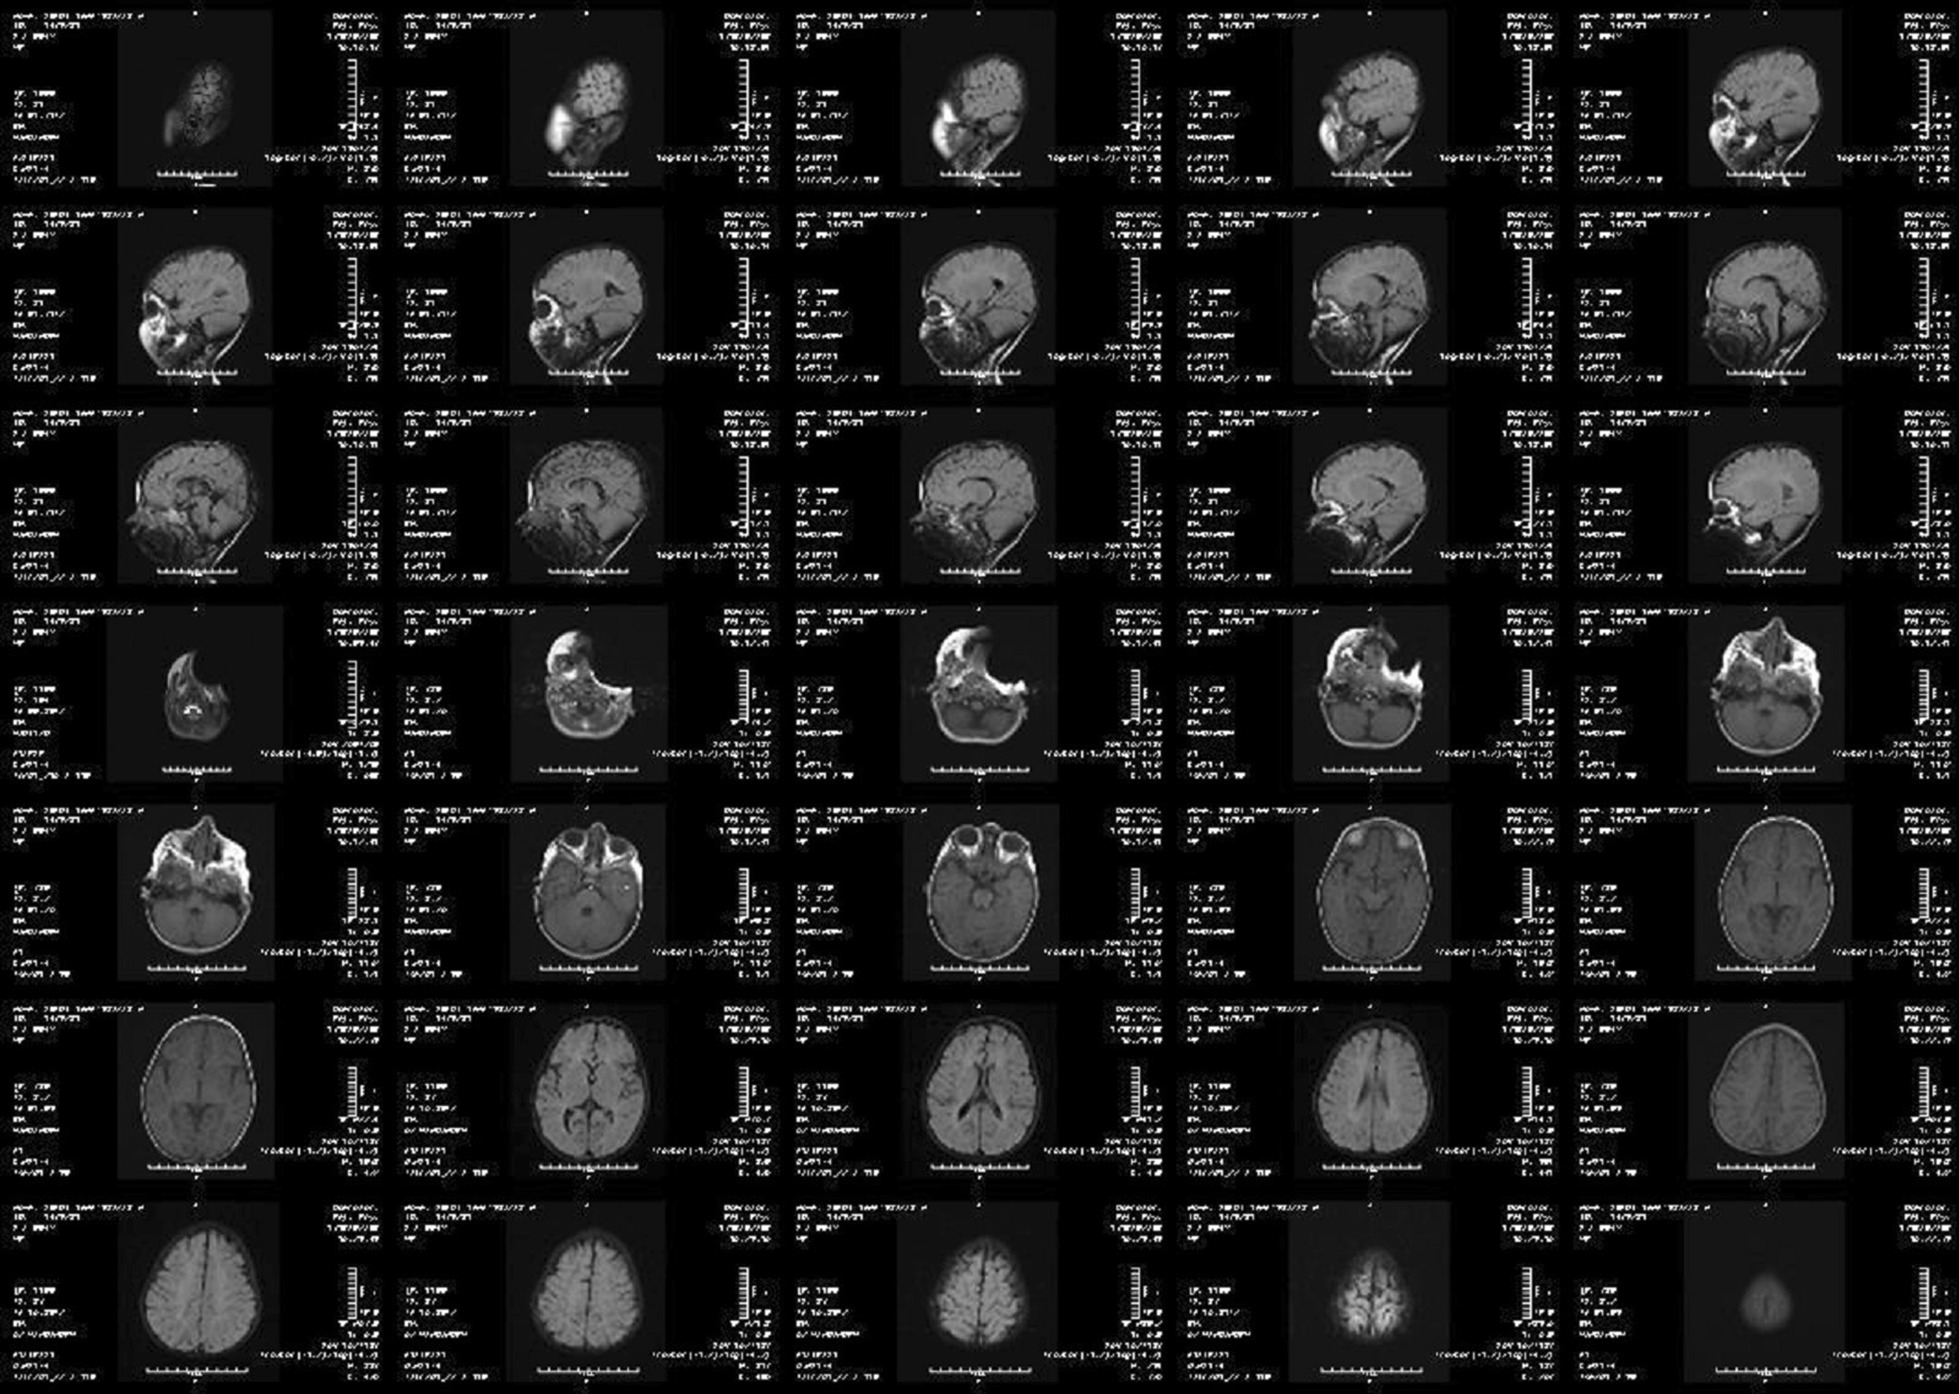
Brain MRI scan shows that all parameters were normal except mildly delayed myelination and nonspecific periventricular hyper-signal intensity
